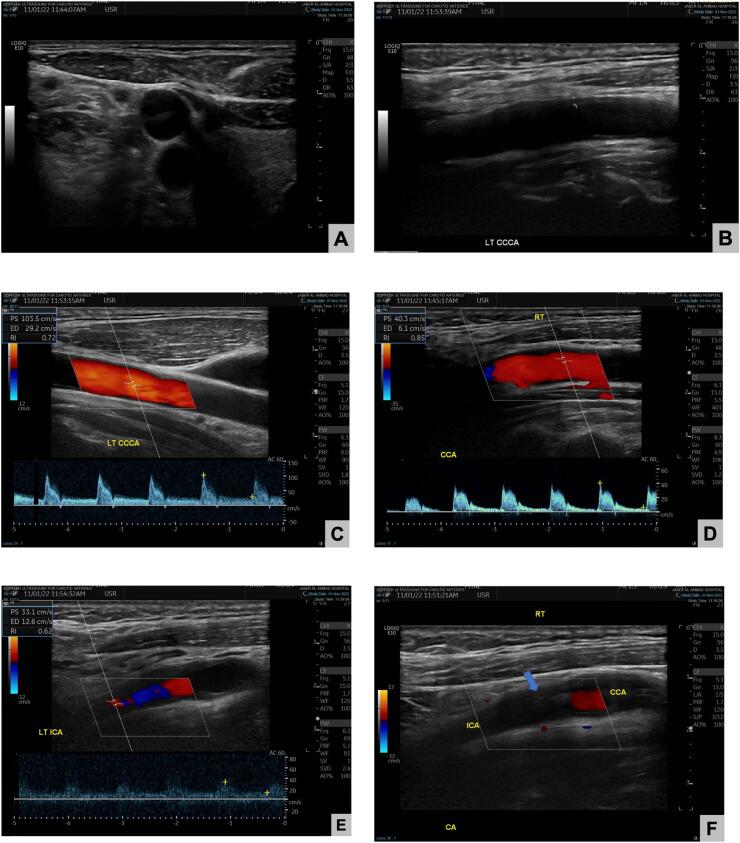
Carotid doppler scanning: A: Patent common carotid artery B: Patent left common carotid artery C: Normal left common carotid artery with normal waveforms and anterograde flow D: Retrograde flow of the right common carotid artery according to the carotid doppler. E: Average velocity along the carotid arteries and no areas of abnormal high velocity F: ICA Stump in the right ICA.
